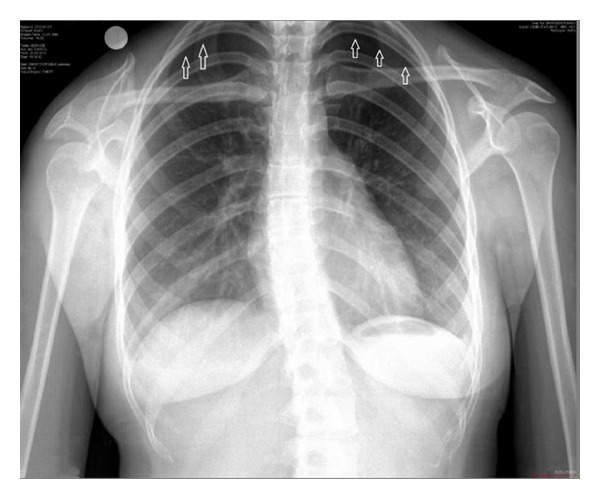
Chest X-ray shows bilateral small pneumothoraces. White arrows indicate pleural lines.
